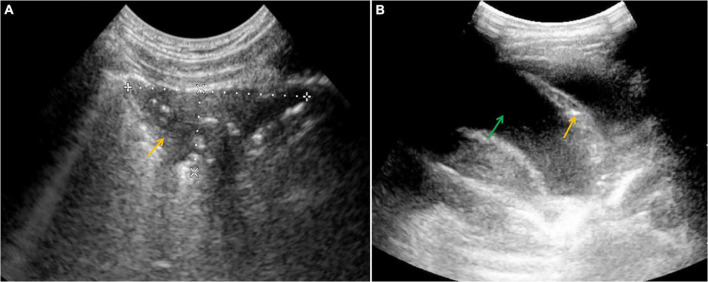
Ultrasound (US) examination of the lung, convex probe, (A) an area of subpleural consolidation (marked with an arrow), (B) pleural effusion (a green arrow shows the fluid and an orange one shows atelectasis).
